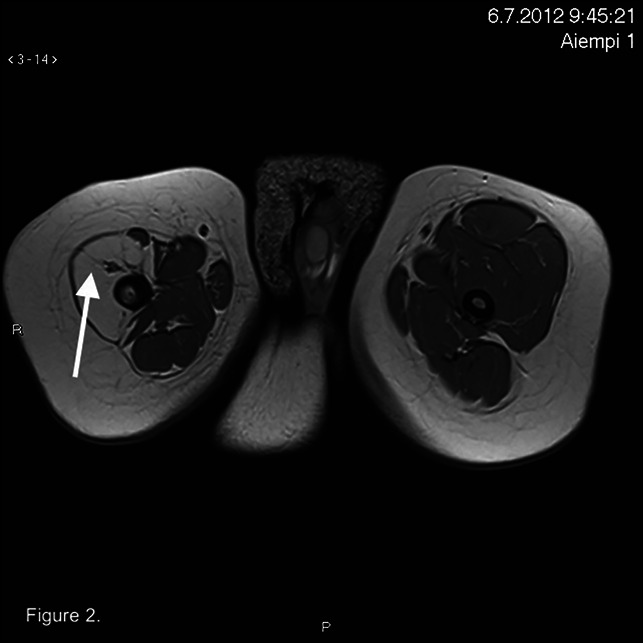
MRI image showing an arrow pointing to the missing quadriceps femoris muscle.
